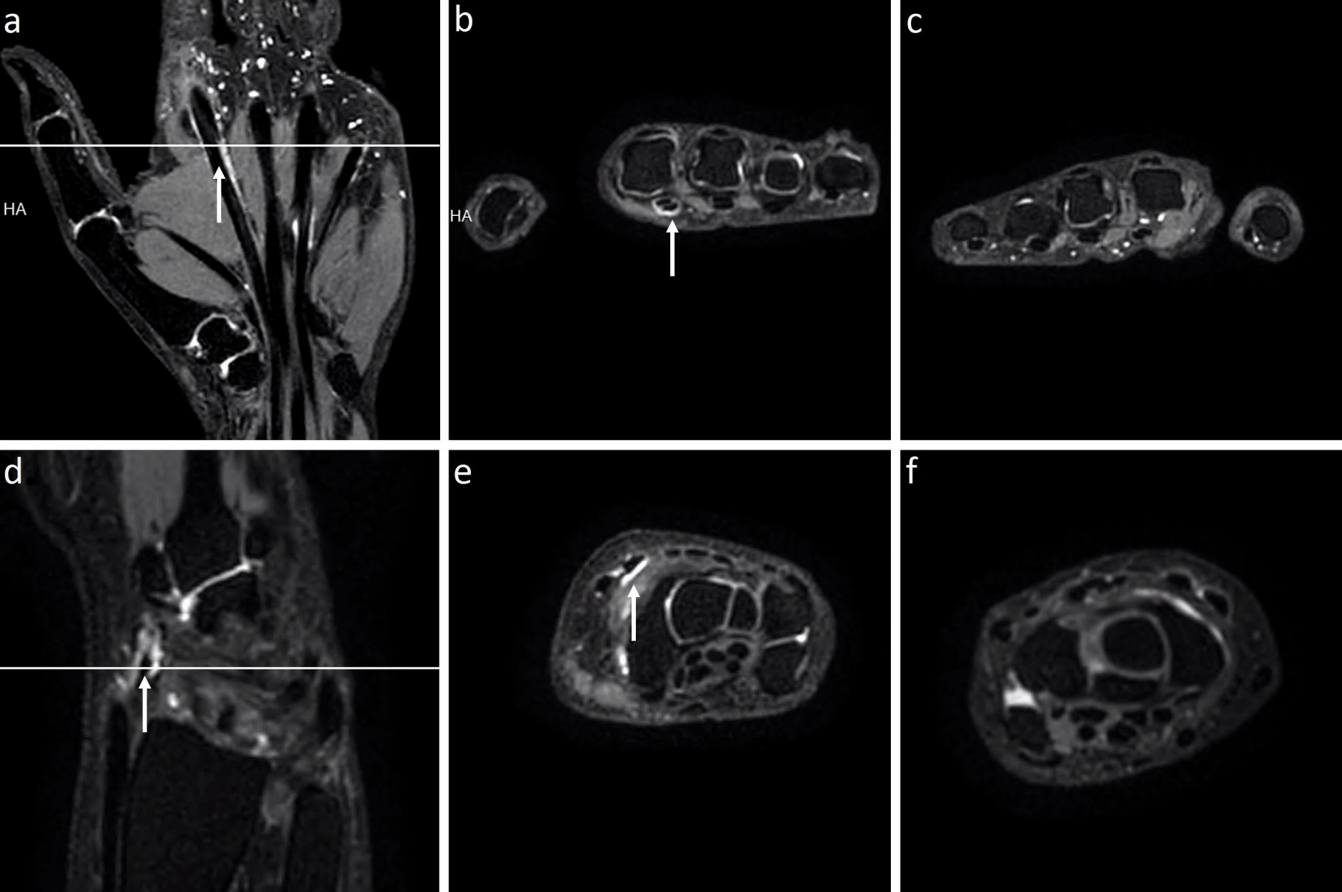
An mDixon MRI example showing unilateral tenosynovitis in a patient with CSA at two locations. A 48-year-old woman with unilateral tenosynovitis on water-only mDixon MRI at two locations. (a,d) Coronal and (b,e) axial slices of the left hand at two levels. (c,f) Axial slices at corresponding levels of the right hand. Tenosynovitis was scored in the flexor digitorum tendon at the left second metacarpophalangeal joint and in the extensor carpi radialis longus and brevis (indicated by the white arrows). A multiloculated ganglion cyst was observed in the right hand, and no subclinical inflammation was present. CSA, clinically suspect arthralgia; mDixon MRI, modified Dixon MRI.
